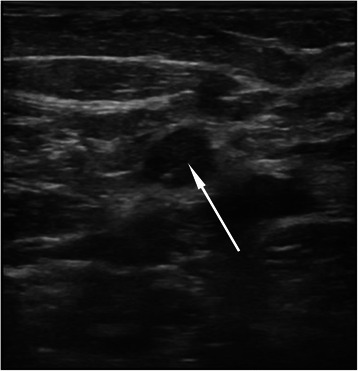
Ultrasound localization of suspicious lymph nodes 2 hours before SLNB. The red arrow shows the hook wire.
